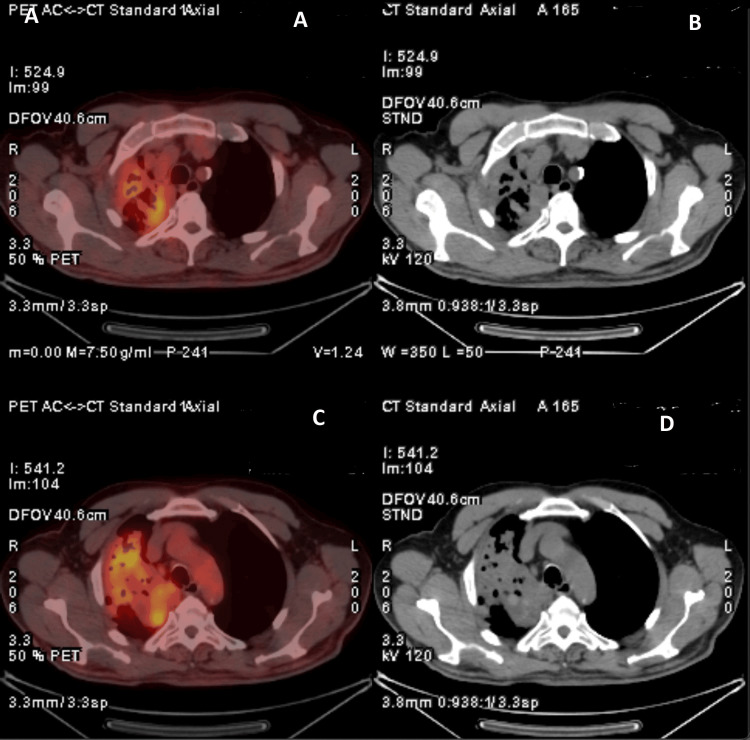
PET-CT Image Demonstrating Intense Fluorodeoxyglucose (FDG) Uptake (A, C) Axial 18F-FDG PET/CT fusion images demonstrating areas of increased metabolic activity within the thoracic lesion. (B, D) Corresponding axial non-contrast CT images at the same anatomical levels showing the structural correlates of the metabolically active regions. The combined assessment of PET and CT images enables simultaneous evaluation of metabolic and anatomical characteristics of the lesion.
